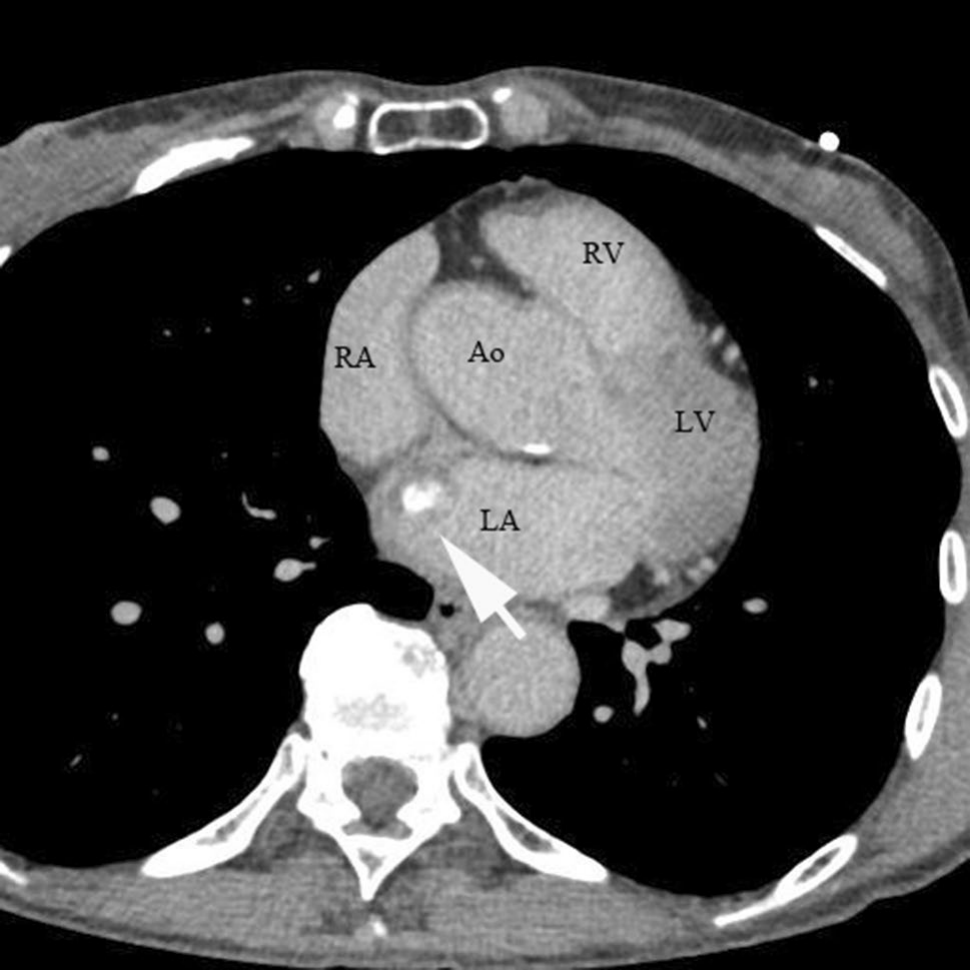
Enhanced computed tomography demonstrated partly calcified tumor at the left atrial wall (arrow). *RA* right atrium, *LA* left atrium, *Ao* aorta, *RV* right ventricle, *LV* left ventricle
